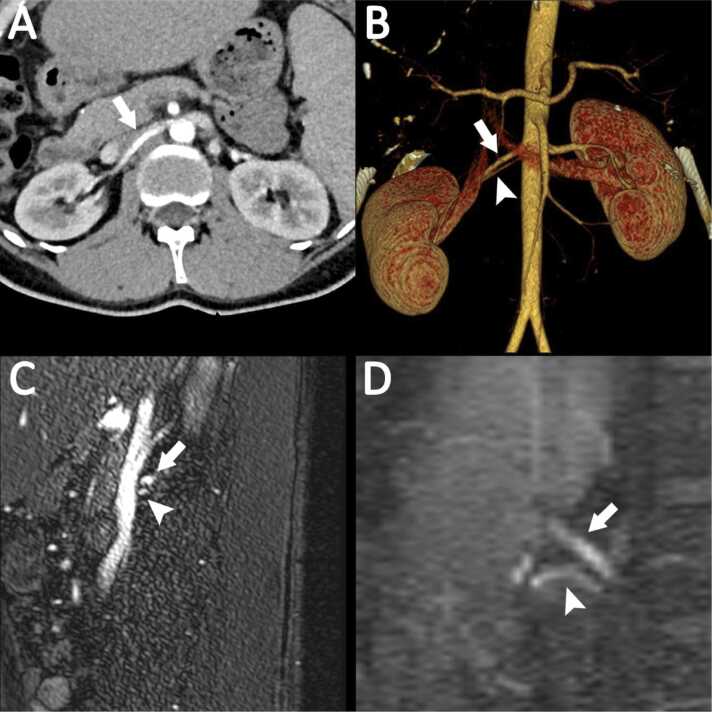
76 year-old male with accessory right renal artery coursing underneath the main renal artery (arrow), which is not clearly detectable on axial slices of the dual-energy CTA (A), however visible (arrowhead) on the coronal MIP CTA reconstruction (B). Right renal artery (arrow) and accessory renal artery (arrowhead) are detected reliably on sagittal slices of QISS MRA (C) as well as on the QISS MRA coronal acquisitions (D).
